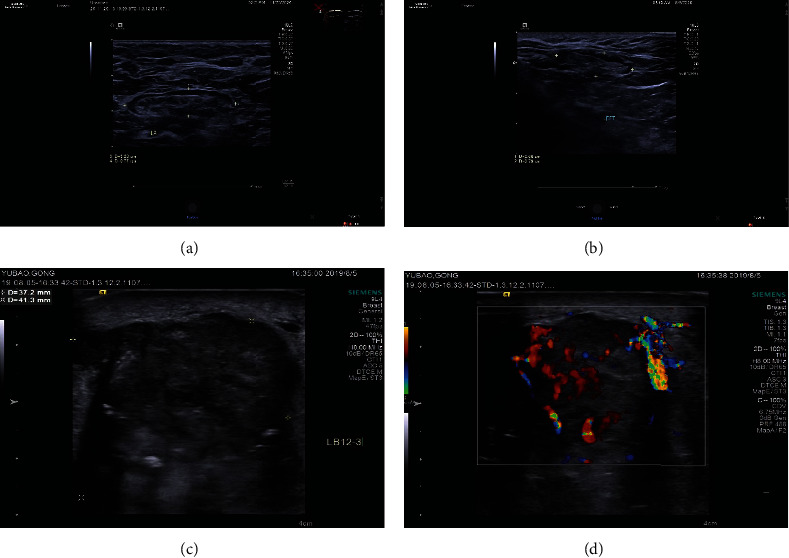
Ultrasound signs for different axillary lymph node statuses.
